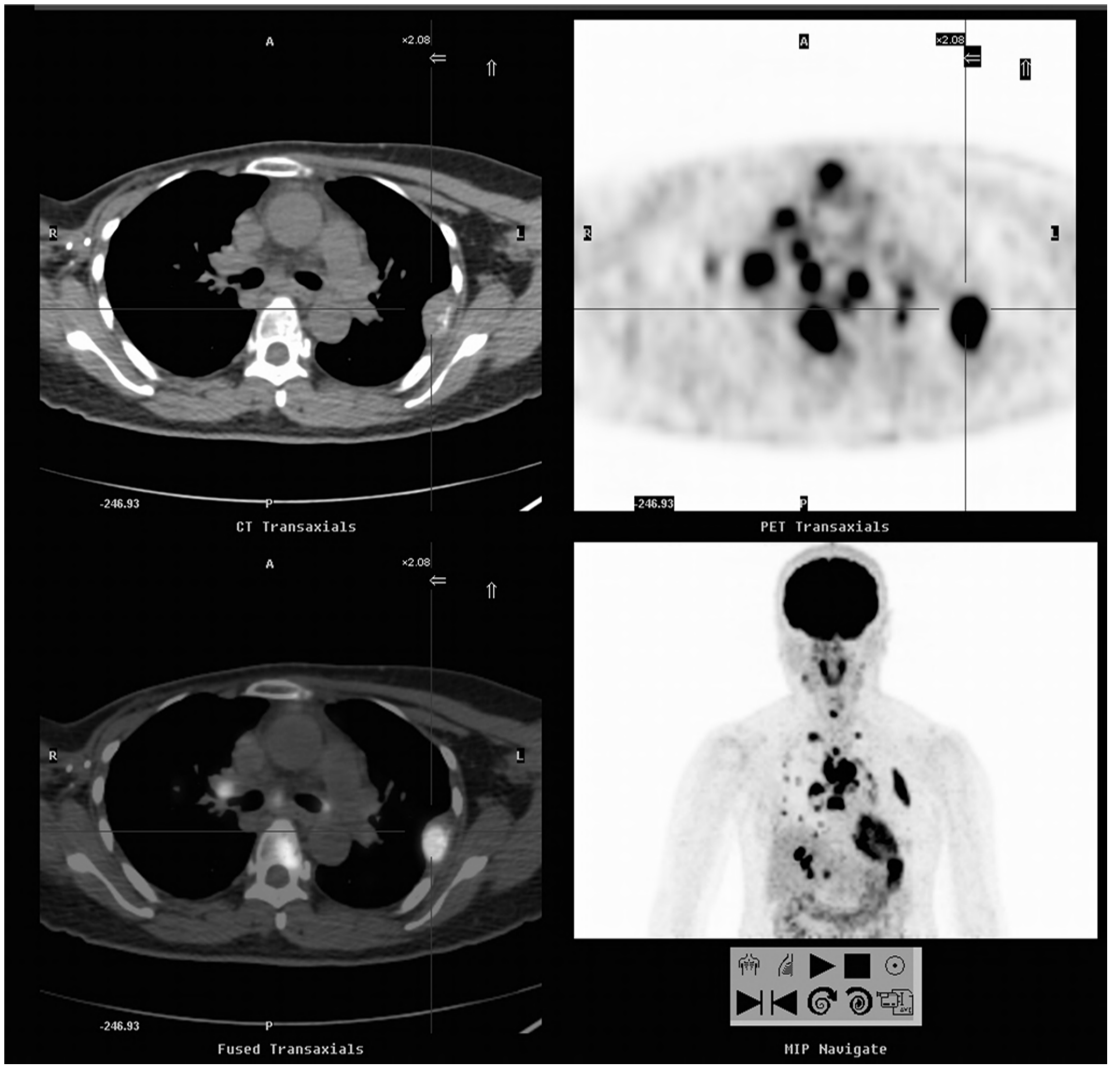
A 55-year-old woman with a history of breast cancer (invasive ductal carcinoma of the right breast, stage I) underwent a FDG-PET/CT scan due to elevated serum CA15-3 level of 44.7 U/ml. FDG-PET/CT showed local recurrence in right anterior chest wall, extensive lymphatic invasion, and multiple metastases to liver, lungs and bones (SUVmax = 17.5, cross cursor). The patient's disease progressed rapidly, and she expired 8 months later. FDG-PET/CT, 2-[^18^F]fluoro-2-deoxy-Dglucose-positron emission tomography/computed tomography; SUV, standardized uptake value.
